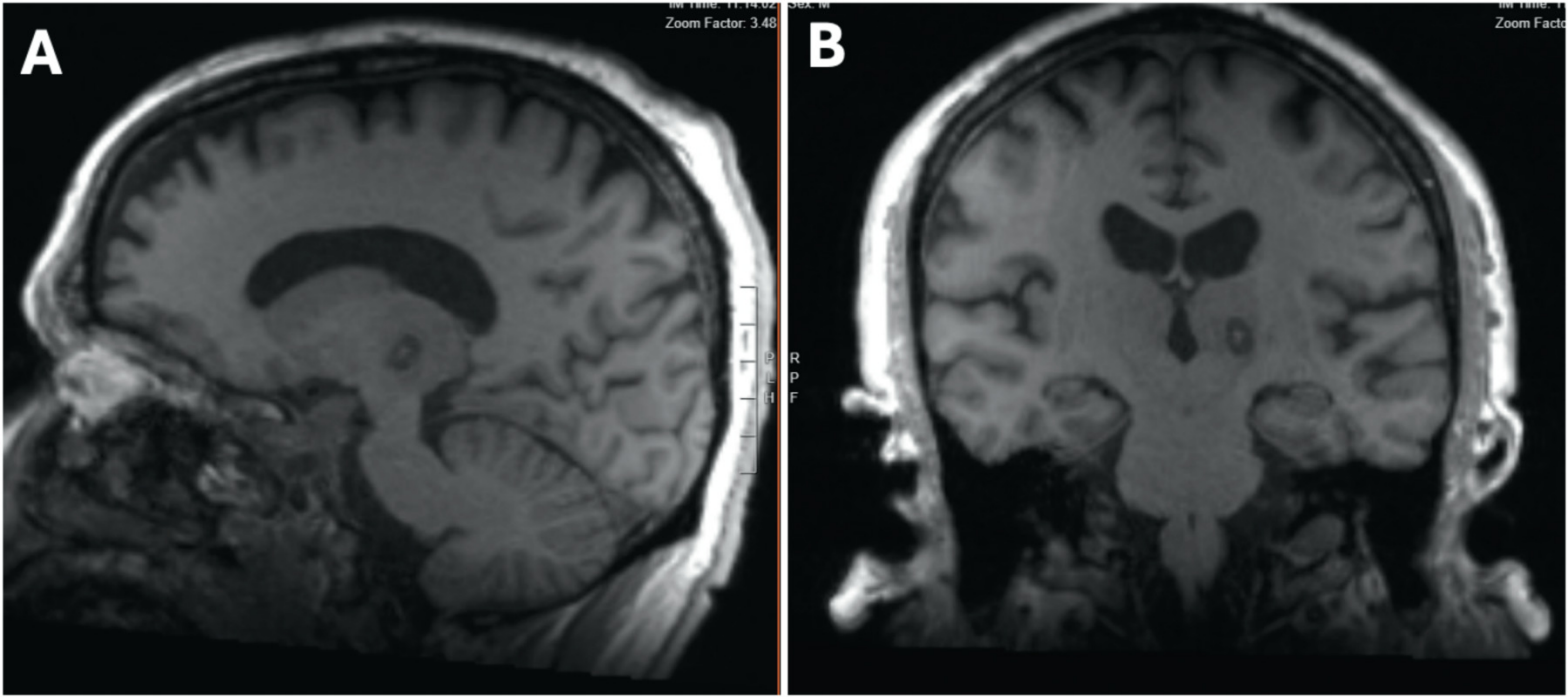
T1w postoperative MRI showing the conformational behavior of the standard lesioning strategy. Notably, on the sagittal view, the lesion is tilted in an oblique posterior angle (A) and in an oblique medial angle on the coronal view (B), which do not follow the anatomical orientation of the Vim.
